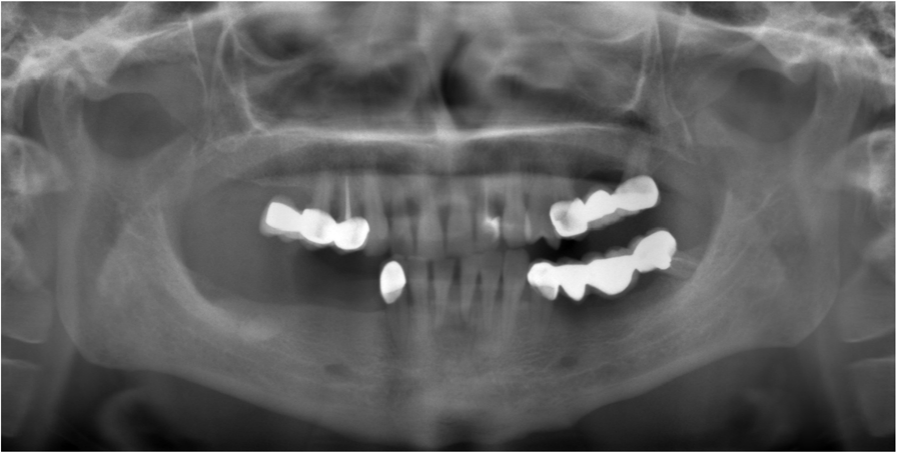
Panoramic X-ray evaluation of patient
